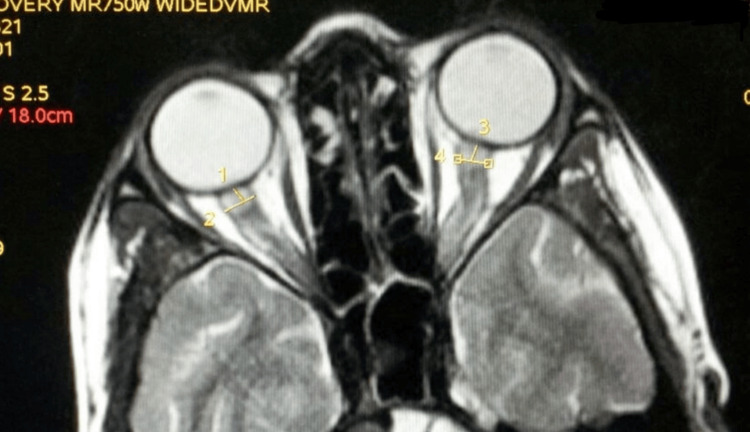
ONSD measurement at 3mm depth by electronic caliper in axial segment of MRI ONSD: optic nerve sheath diameter; MRI: magnetic resonance imaging
